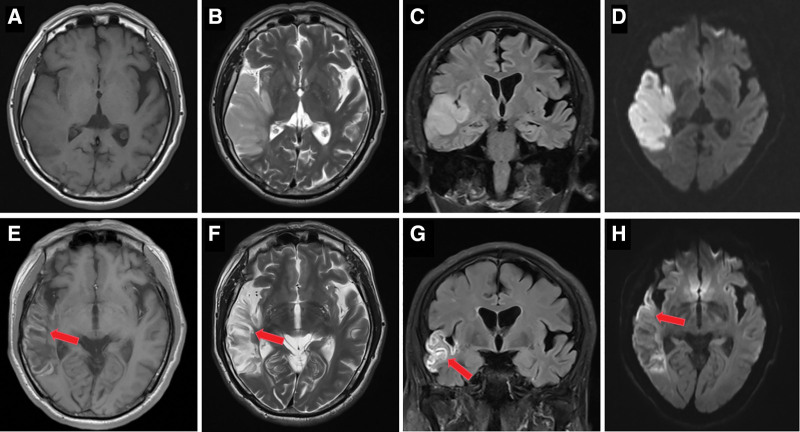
A 62-year-old man with left temporal infarction. (A–D) Images obtained at 4 days after onset, (E–H) at 35 days after onset. (A) T1WI, (B) T2WI, and (C) FLAIR do not show high signals along the cortex. (D) DWI shows laminar restricted diffusion in the right temporal lobe. (E) T1WI, (F) T2WI, (G) FLAIR, and (H) DWI from the corresponding sections show hyperintensity along the temporal gyri during follow-up which is typical of CLN. (CLN is indicated by red arrows). CLN = cortical laminar necrosis, DWI = diffusion-weighted imaging, FLAIR = fluid-attenuated inversion recovery, T1WI = T1-weighted imaging, T2WI = T2-weighted imaging.
